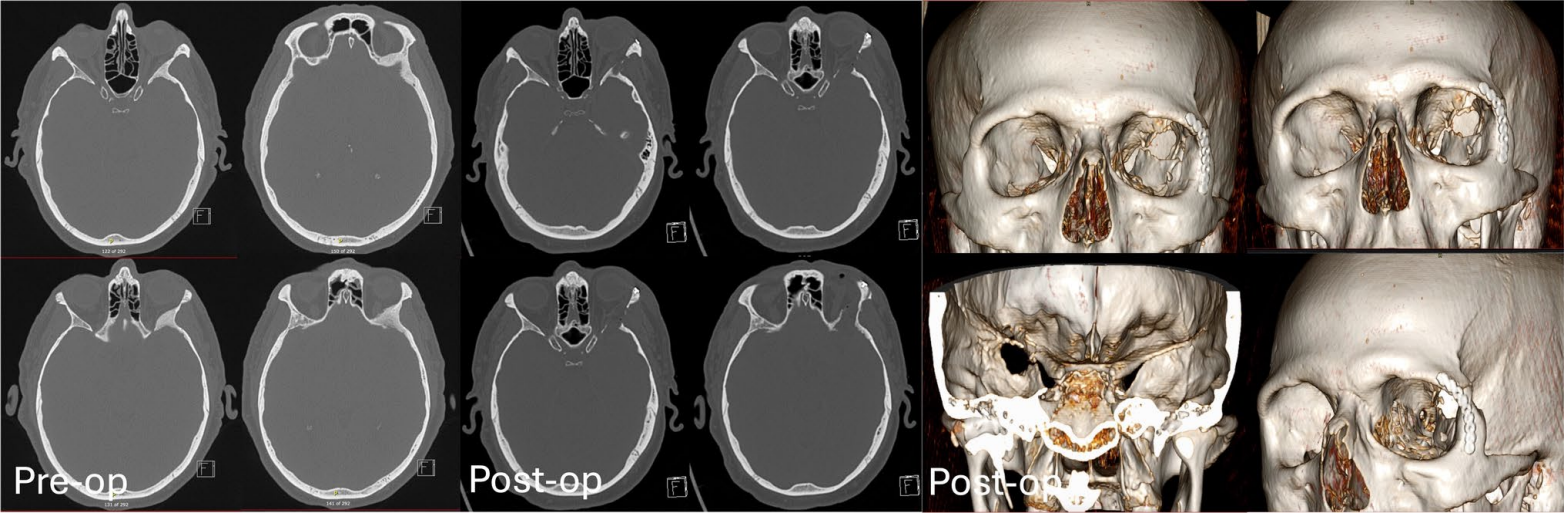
Pre- and post-operative CT with bony reconstructions demonstrating bony removal and tumour resection
